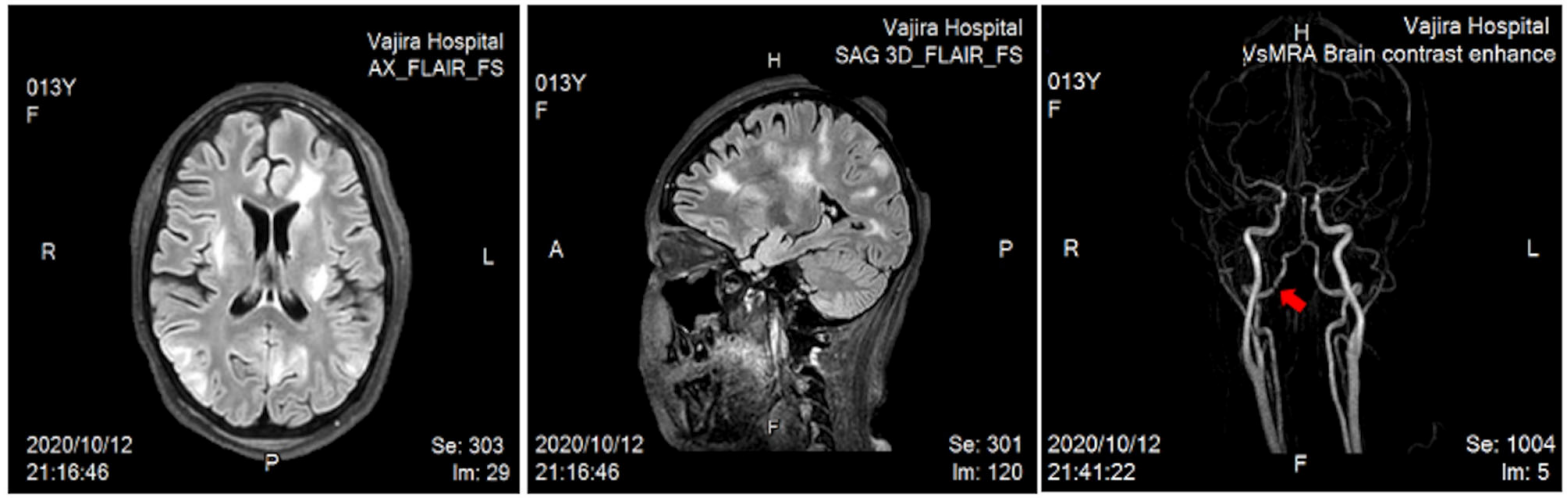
Magnetic resonance imaging of the brain revealed multifocal patchy T2/FLAIR-hyperintense lesions in the subcortical and deep white matter of the bilateral fronto–parieto–occipito–temporal lobes, posterior limb of the left internal capsule, and superior cerebellar vermis. Bilateral ACAs, MCAs, and PCAs were unremarkable, but magnetic resonance angiography showed irregular narrowing along the V4 segment of the right vertebral artery (arrow). ACAs, anterior cerebral arteries; MCAs, middle cerebral arteries; PCAs, posterior cerebral arteries.
